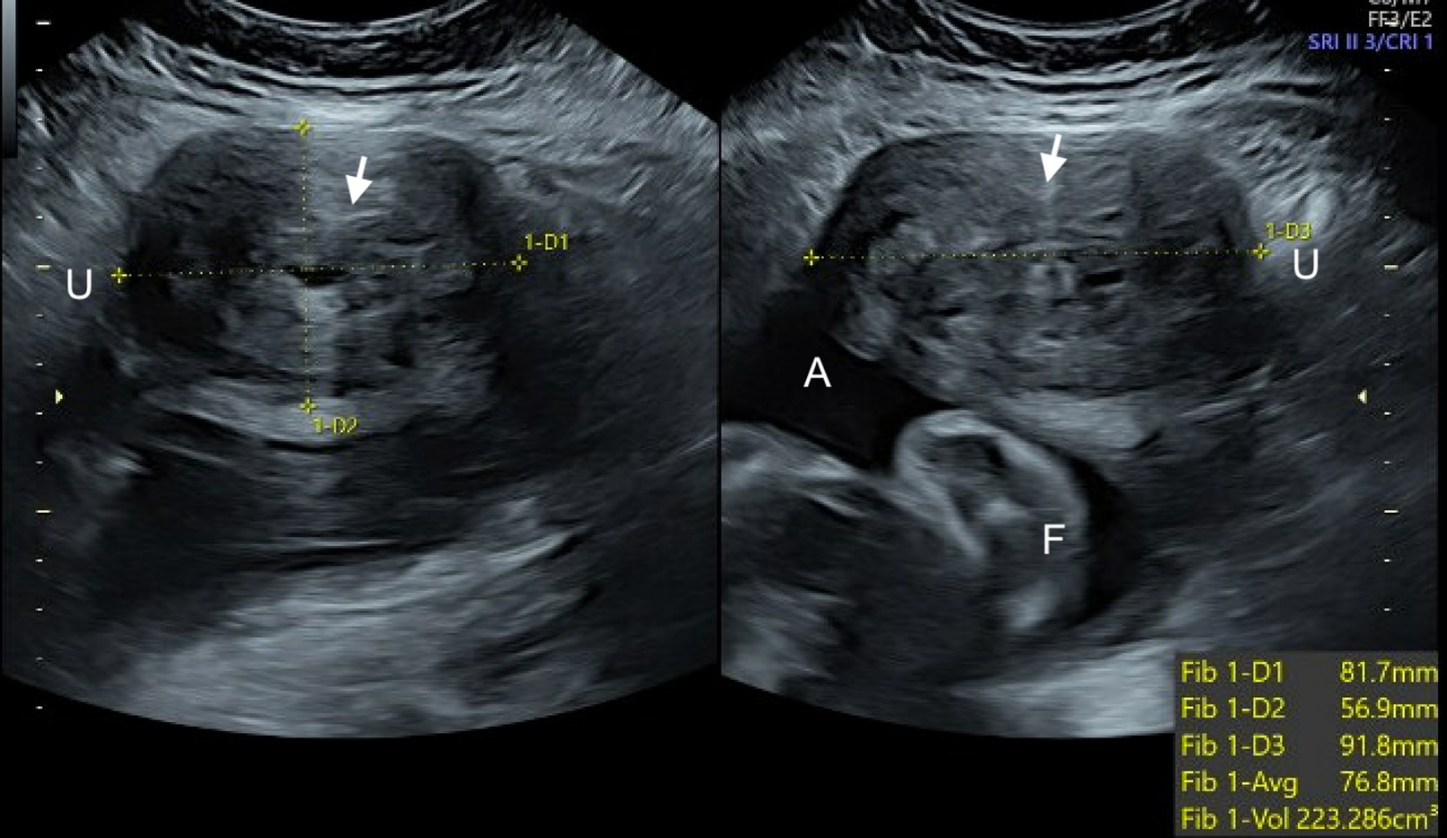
Prenatal ultrasound at 30 weeks of gestation showing a larger uterine fibroid (arrow), uterine wall (U), fetal parts (F), and amniotic fluid (A).
